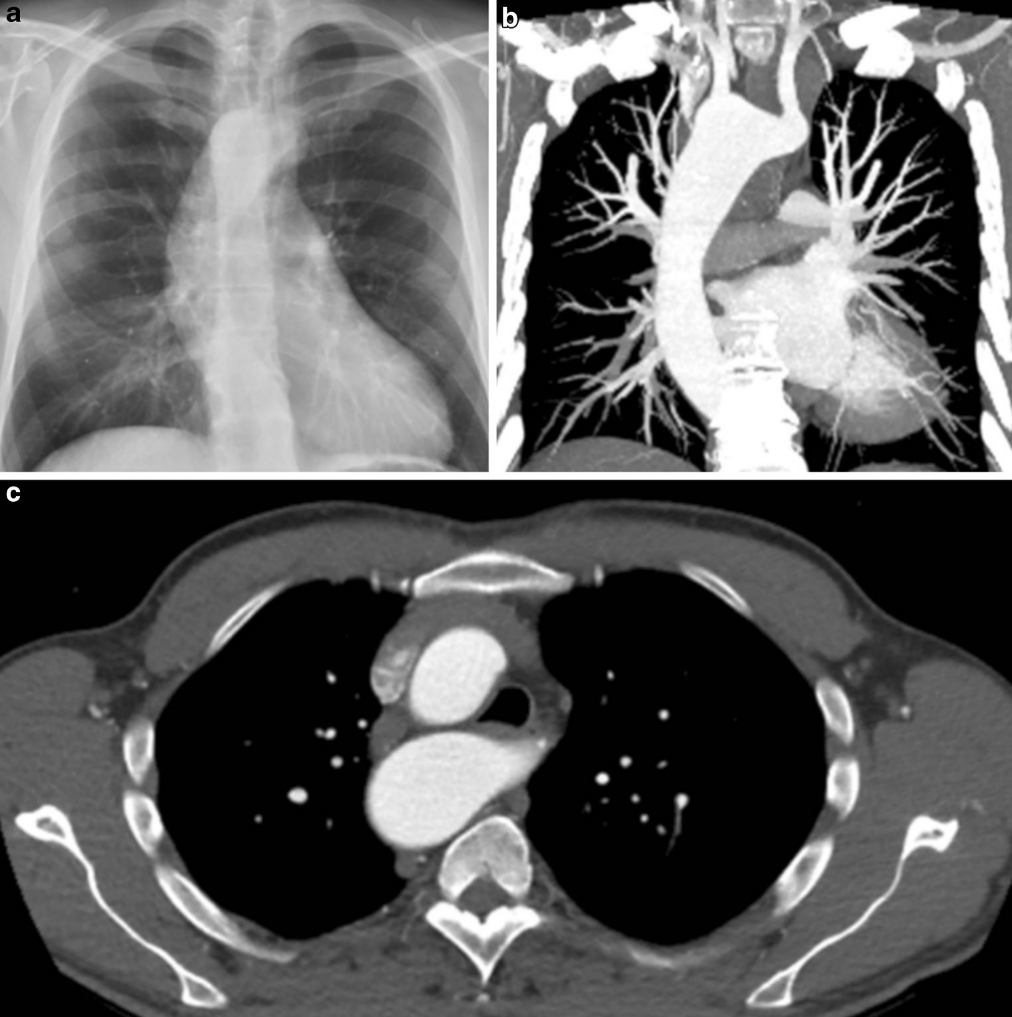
An anteroposterior chest radiograph (a) and the corresponding frontal plane (b) and transverse plane (c) CT images of the patient described
